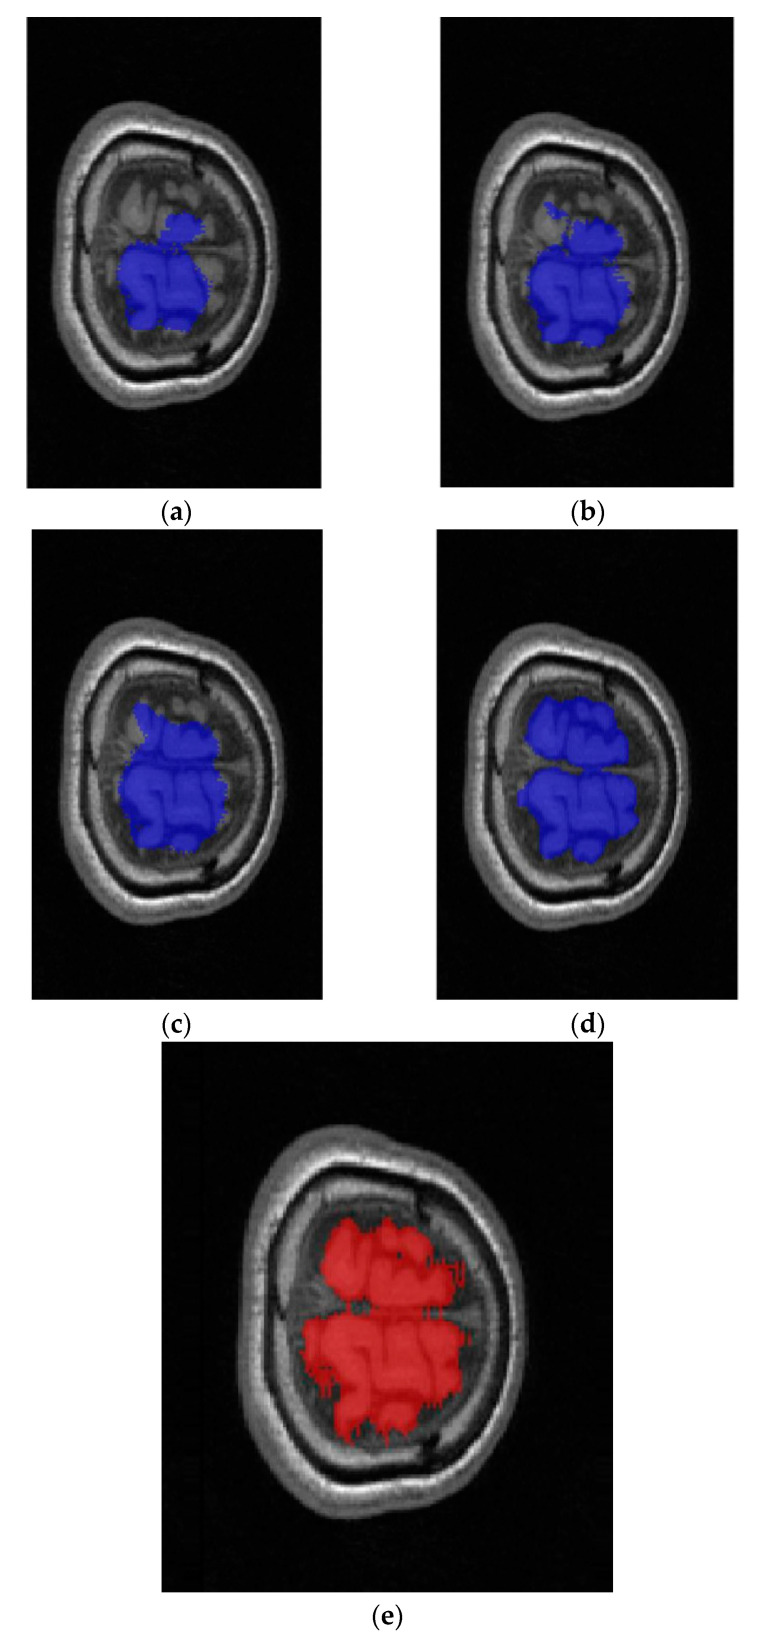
Predicted masks overlaid on MRI data (transverse section). (a) The brain mask predicted using the original U-Net model. (b) The improvement in prediction performance achieved through K-means preprocessing. (c) The enhancement in the edge area achieved by employing a hybrid net. (d) The good performance obtained using the PHC-U-Net model. (e) The superimposed reference label mask on the MRI data.
